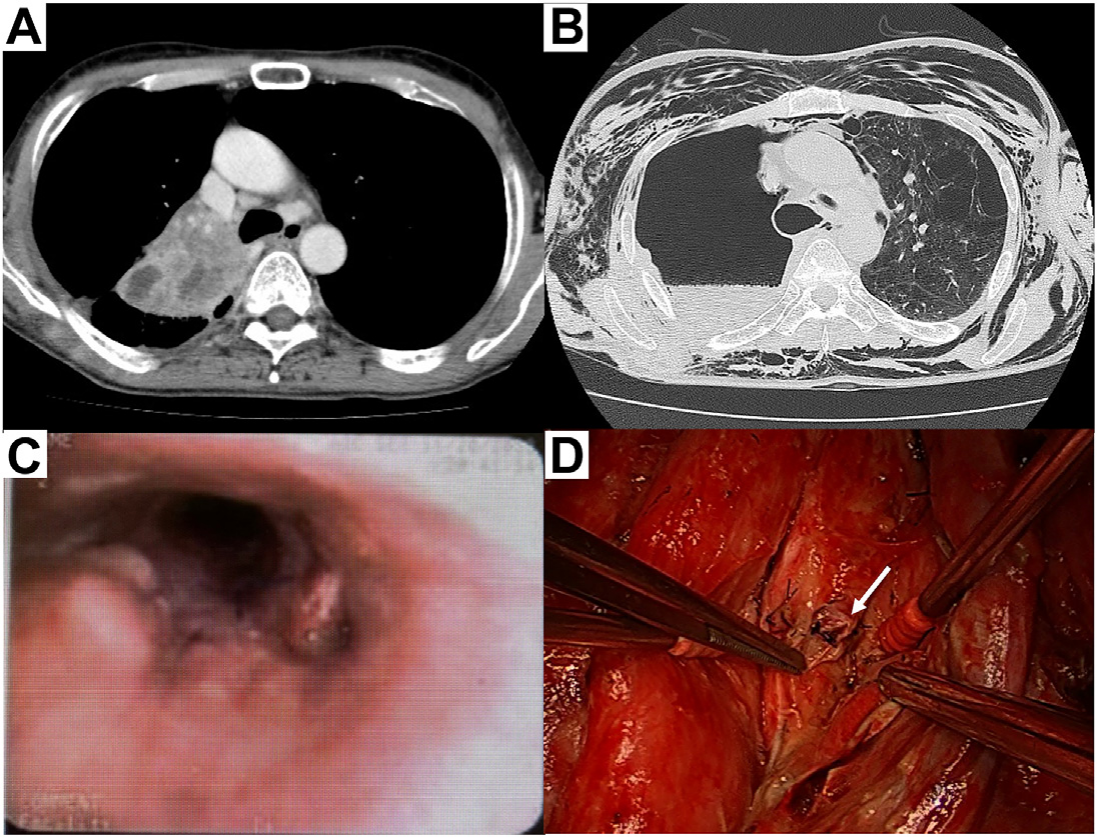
(A) Preoperative computed tomographic findings of a primary lung tumor in a 58-year-old man who underwent right sleeve pneumonectomy for lung squamous cell carcinoma (cT3 N1 M0). (B) Computed tomographic findings of subcutaneous emphysema exacerbation on the day after operation. (C) Bronchoscopic findings at the anastomosis between the trachea and the left main bronchus the day after operation. A fistula was observed between the right side of the cartilage and the membranous part of the anastomosis. (D) Intraoperative anastomotic findings during reoperation for the bronchial anastomotic fistula. One anastomotic thread on the right lateral wall of the cartilage at the anastomotic site (arrow) was broken.
